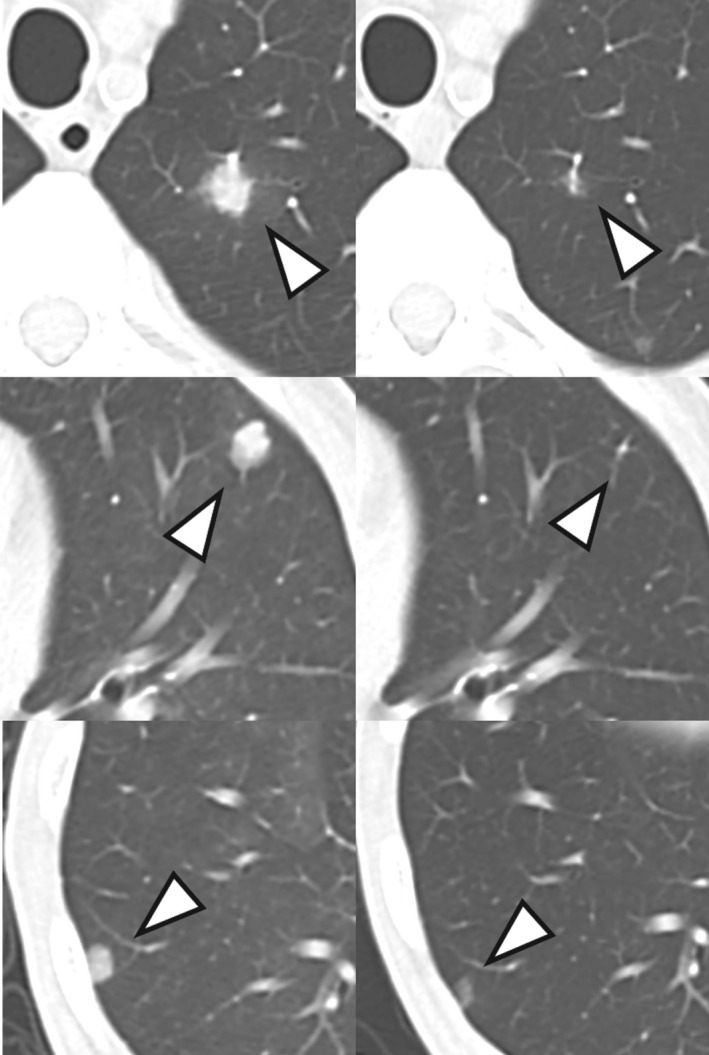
Chest CT before combination therapy with ipilimumab plus nivolumab (left) and after 6 months of treatment (right). The lung metastases have shrunk
